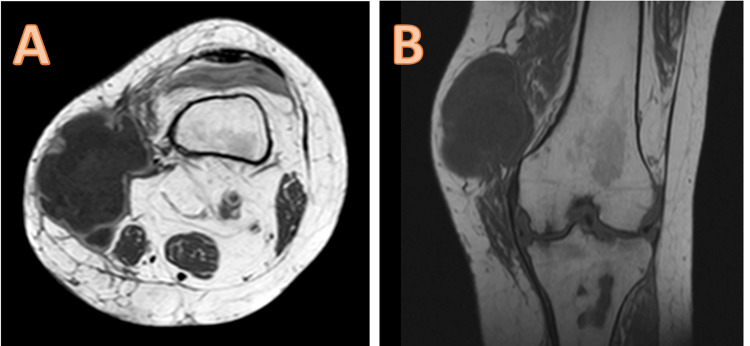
MRI appearance in axial (A) and frontal (B) sections without contrast product injection
